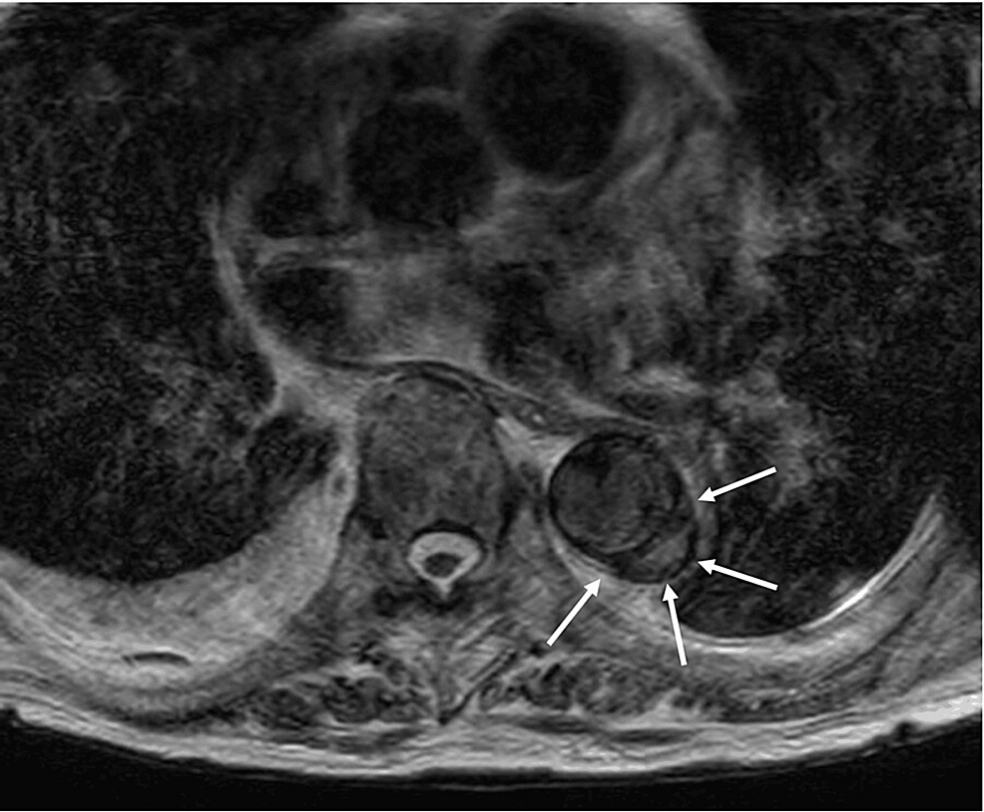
Magnetic resonance imaging of the descending aorta. T2 axial magnetic resonance imaging with white arrows showing a localized descending aortic dissecting aneurysm.
